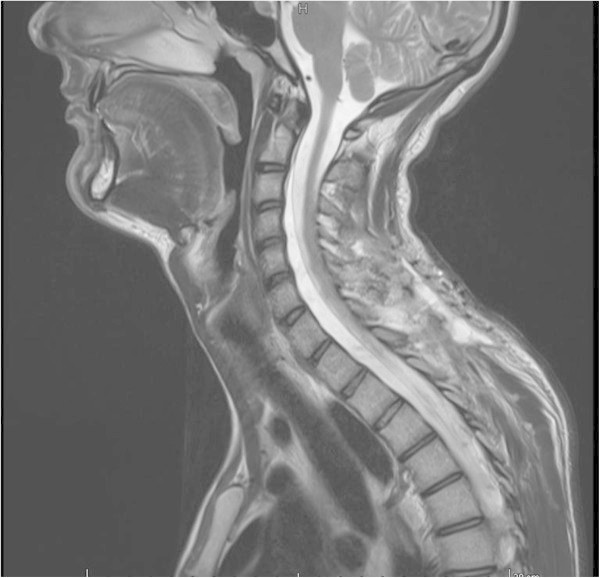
Case II: sagittal- weighted T2 weighted MRI demonstrating a ventral subdural collection at C2- T1.
